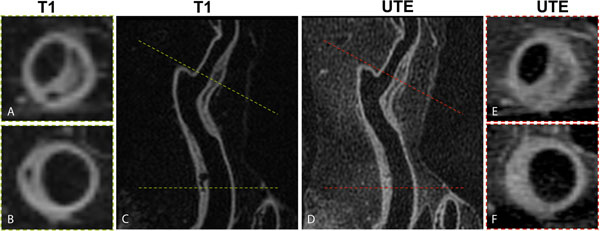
T1 weighted and UTE MRI of a coronary artery with large fibrocalcific plaques. (A, B) T1W MRI at two cross-sections along the coronary artery. (C) T1 weighted multiplanar reformat of the vessel. (D) UTE multiplanar reformat, and (E, F) UTE cross sections of the vessel. Two large plaques are seen along the coronary artery in the multiplanar reformats. Foci of profound T1 hypointensity, which appear isointense on the UTE images, are seen in these plaques consistent with plaque calcification.
